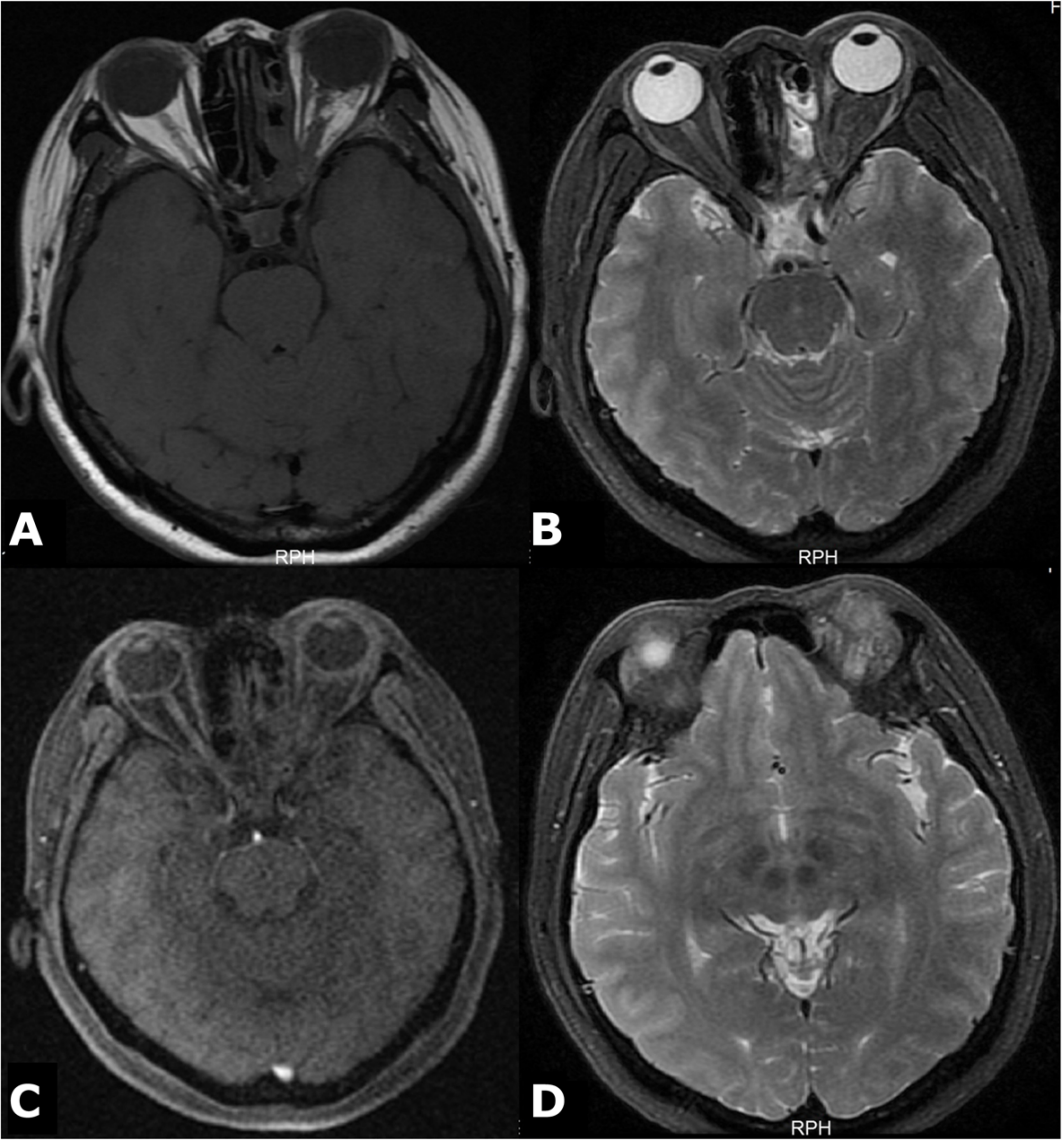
Three months after the surgery, T1-weighted images (a), T2-weighted images (b) and FLAIR images (c) all showed that the patient’s tumour had been excised and the signs of frontal lobe oedema had disappeared on T2-weighted images (d)
